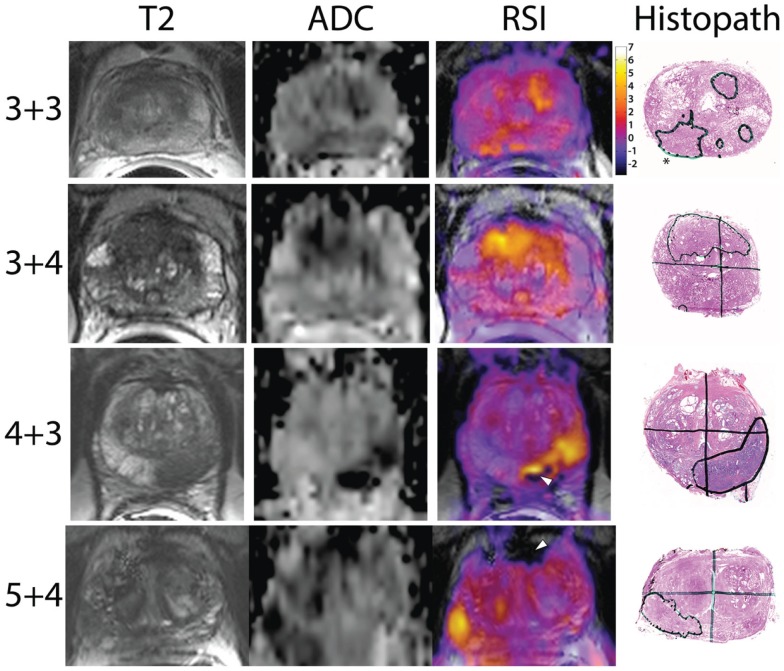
Representative images showing RSI *z*-score maps across Gleason scores: the *y*-axis shows the pathologic Gleason score with the *x*-axis designating the MRI sequence. The last column displays the whole-mount pathology with the corresponding cancer region of interest circled in black. The star in the top right pathologic figure represents the pattern 3 + 3 prostate cancer while the other lesions are 4 + 3. White arrowheads in the higher-grade patients show areas of signal void, which could be interpreted as false positives on the ADC maps.
